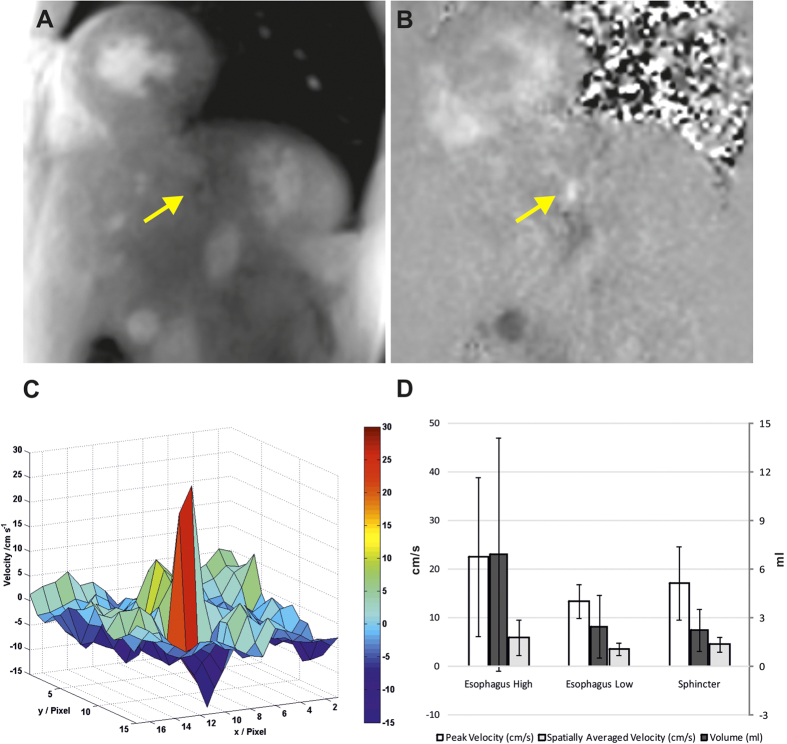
Real-time flow MRI of normal bolus transport through the lower oesophageal sphincter perpendicular to the imaging plane (same subject as in Fig. 1). (A) Magnitude image and (B) phase-contrast flow map (6 mm section thickness, velocity sensitivity 60 cm s^−1^) selected from corresponding real-time flow MRI movies (S7 and S8 in Supplementary Appendix) and (C) corresponding color-coded two-dimensional velocity distribution. Arrows indicate bolus transport through the lower oesophageal sphincter into the stomach. (D) Mean values averaged across 12 healthy subjects for peak velocities and mean velocities spatially averaged over the bolus transport lumen (in cm s^−1^) as well as for flow volumes (in ml) at three different levels along the oesophagus.
